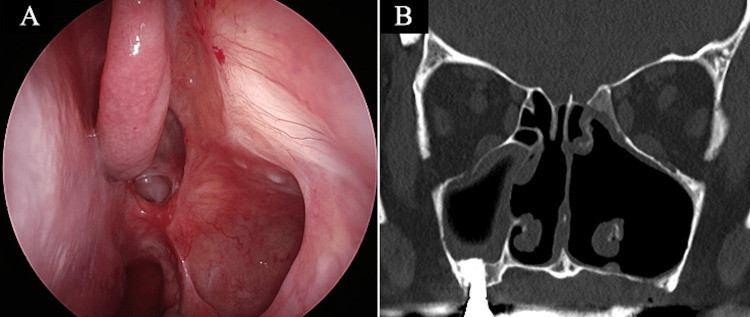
Six-month postoperative findings (A) Nasal endoscopic view; (B) CT scan demonstrating restored aeration of the maxillary sinus
